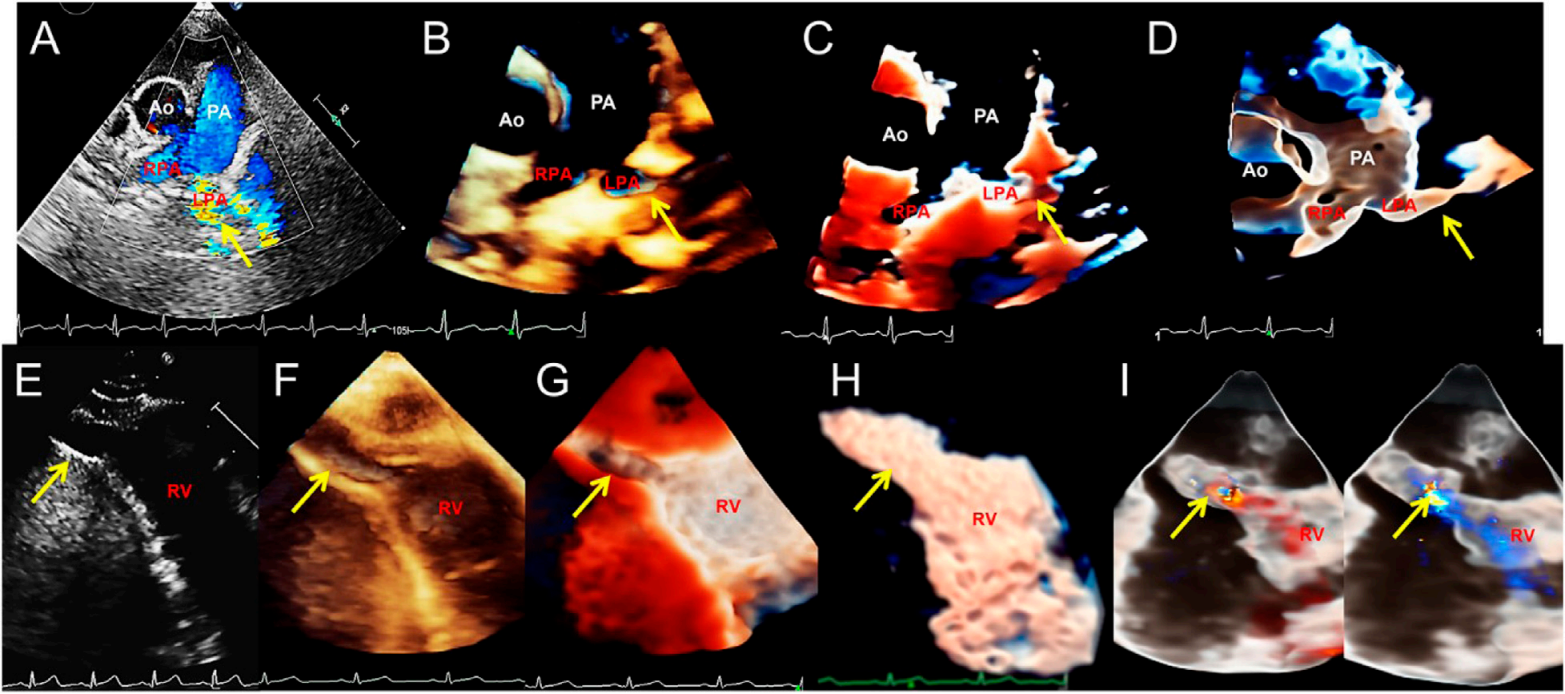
Transthoracic echocardiography shows pulmonary artery sling and right ventricular diverticulum. (A–D). Transthoracic echocardiography showing the pulmonary artery sling on the short-axis section of the great artery. 2D, traditional 3D, TrueVue Light, and TrueVue Glass show abnormal orientation of the left pulmonary artery, which originate from a lower position, bypassing the rear of the trachea and then reflexed to the left, with local stenosis showing increased blood flow speed (arrows). (E–H). 2D, traditional 3D, TrueVue Light, and TrueVue Glass show the diverticula (the end-systolic volume is 15 mm × 9 mm × 9 mm, and the end-diastolic volume is 24 mm × 14 mm × 14 mm, arrows) at the apex of the RV (arrows). (I). TrueVue Glass with color Doppler shows that blood in the right ventricle enters the diverticulum during diastole (left), and blood flow in the diverticulum returns to the right ventricle during systole (right). 2D, two-dimensional; 3D, three-dimensional; Ao, aorta; PA, pulmonary artery; LPA, left pulmonary artery; RPA, right pulmonary artery; RV, right ventricle.
